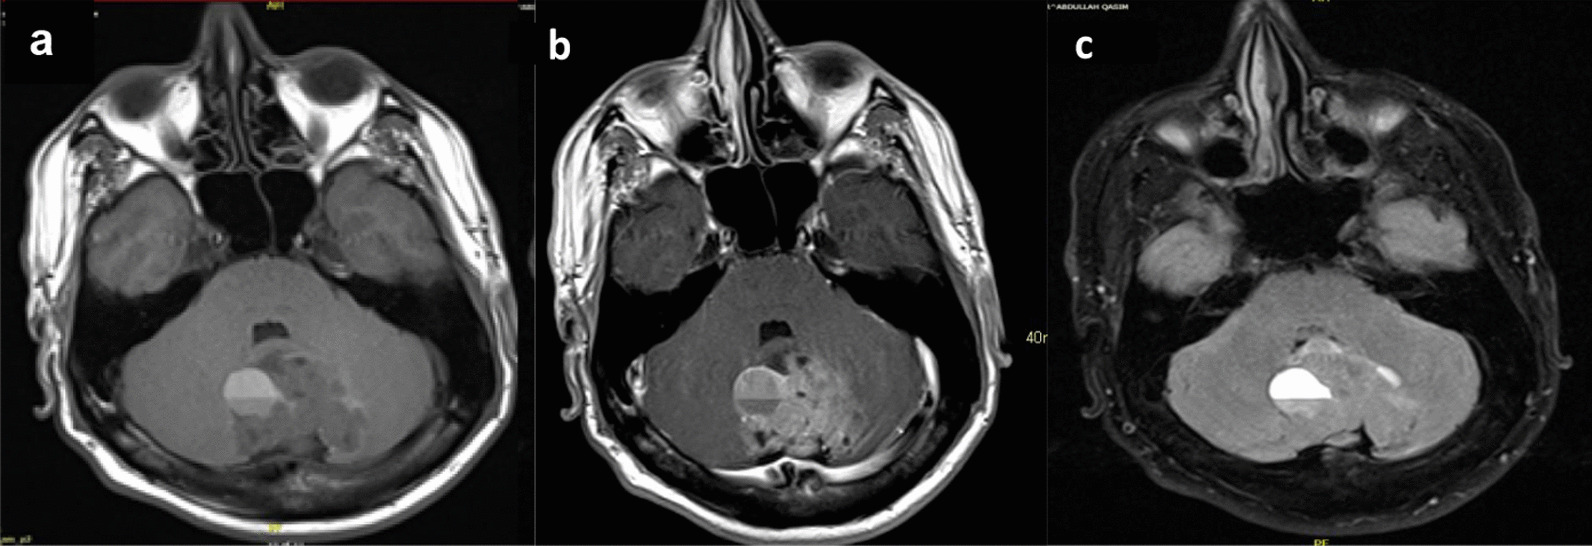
a axial non-enhanced T1, b axial enhanced T1 weighted image and c. axial FLAIR image of the brain. There is a predominantly T1 hypointense mass along the vermis, containing areas of T1 hyperintense fluid–fluid level. It shows strong heterogenous enhancement after contrast administration (b). There is no significant surrounding edema on FLAIR (c). It is compressing and displacing the fourth ventricle anteriorly
